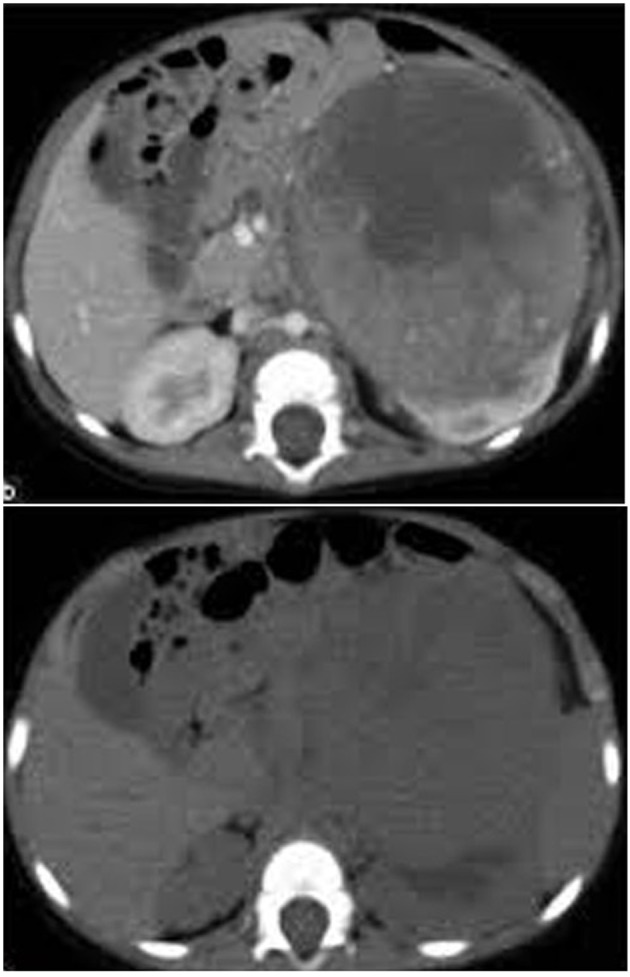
CT image showing a left renal mass with enhancing renal parenchyma posteriorly. Lobulated tumor thrombus is present in the left renal vein and IVC.
